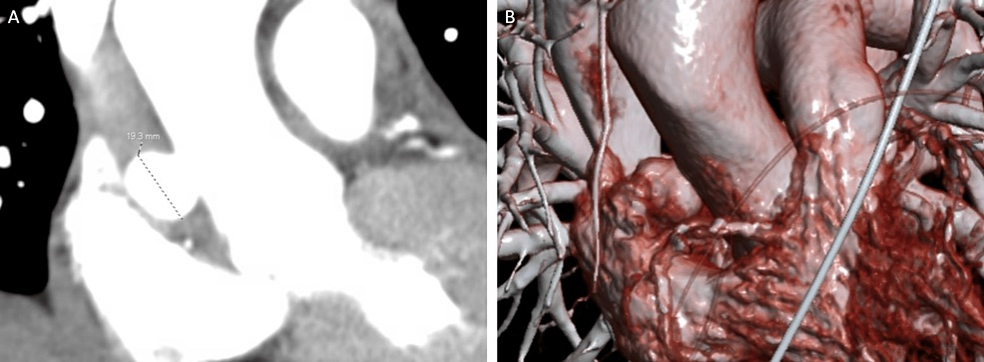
Initial Imaging. CT scan (A) and 3D reconstruction (B) of the chest showing a 1.9 x 1.8 x 1.7 cm proximal ascending aortic pseudoaneurysm.
